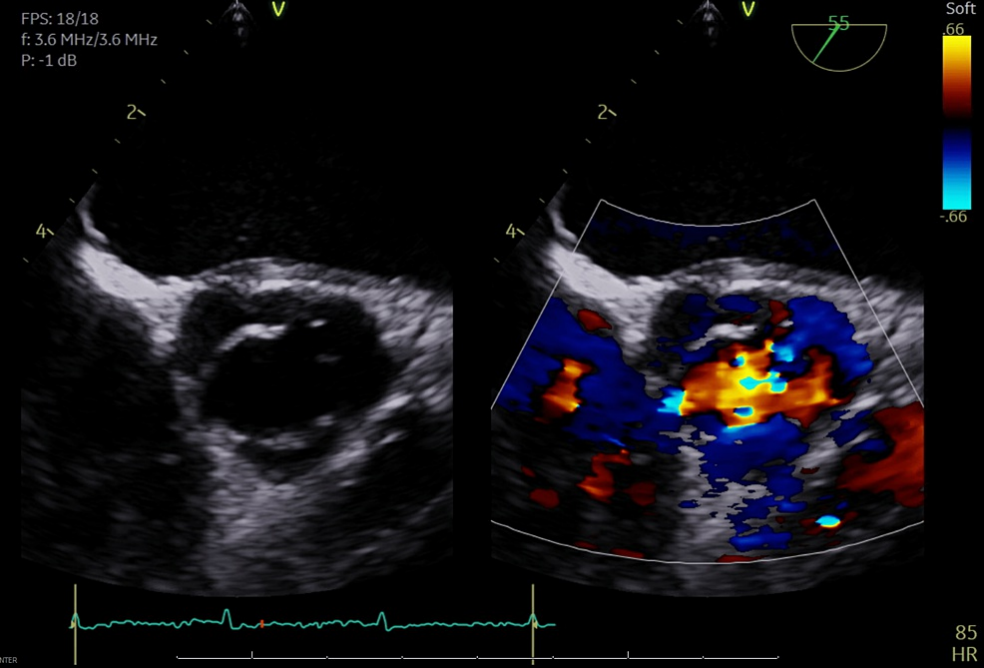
Preoperative echocardiographic depiction of the aortic valve. Preoperative echocardiographic imaging of the aortic valve reveals a trileaflet structure. The echocardiogram shows no significant evidence of aortic stenosis or aortic regurgitation.
